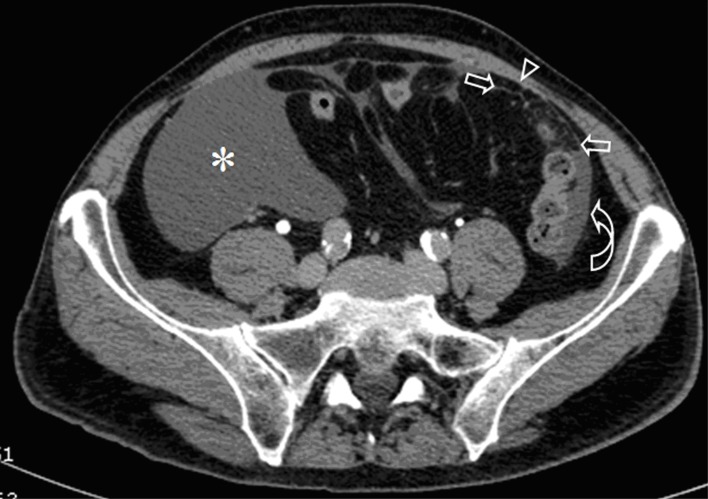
Contrast-enhanced CT of the abdomen shows abundant peritoneal fluid in the right iliac fossa (*) and paracolic fluid in the left side (curved arrow). Note the thick septa with micronodules in the greater omentum (arrows) and circumscribed enhancement of the thickened peritoneum (arrow head).
